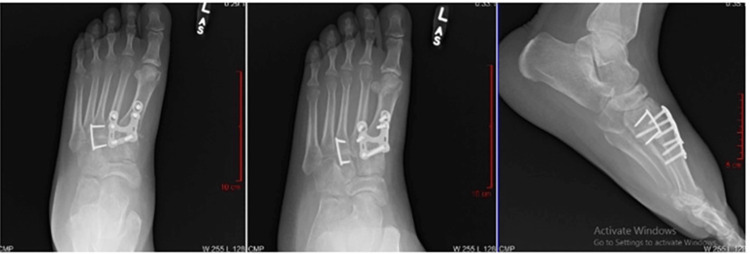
Example of mock patient scenario (Case 5) provided to surgeons; Post-operative radiographs demonstrating midfoot arthrodesis.
